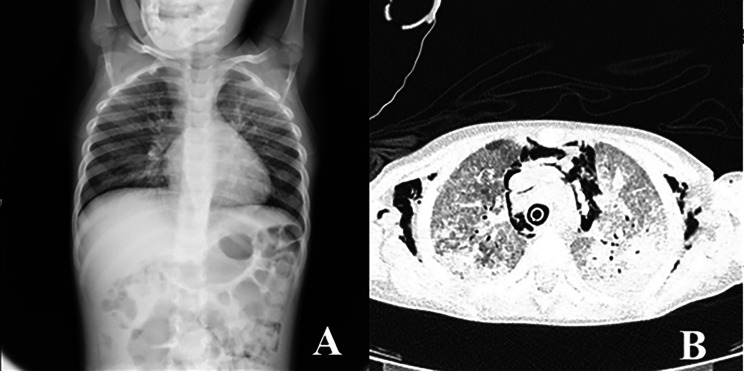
Chest X-Ray (A) and CT (B) showing multiple and bilateral ground glass areas and patchy consolidations in the inferior lobes, pneumomediastinum
